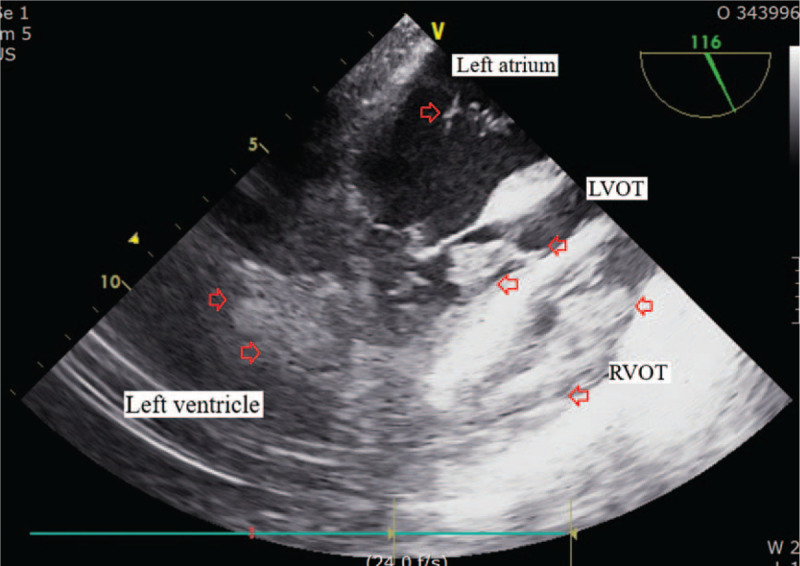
Huge and multiple thromboembolic clots in the left atrium and ventricle, left ventricular outflow tract and right ventricular outflow tract shown by transesophageal echocardiography. Empty arrows indicate thromboembolic clots.
